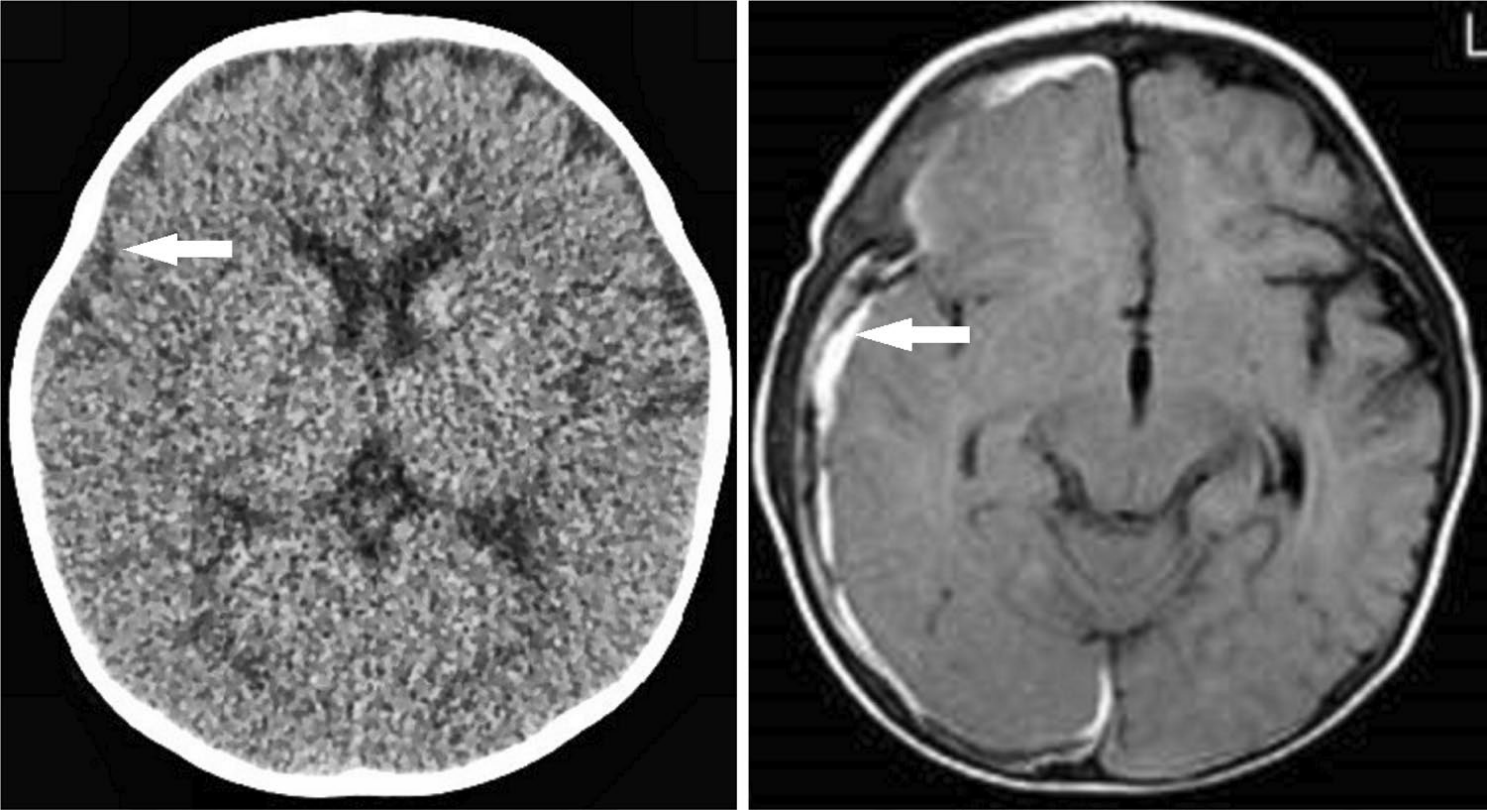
Case 4, male aged 6 months. Left: CT on arrival demonstrating thin, low-density SDH on the right side (arrow). Note that the density of the SDH is higher than that of the cerebrospinal fluid in the lateral ventricles and subarachnoid spaces on the left side. Right: MRI (T1-weighted image) 11 days after symptom onset demonstrating thin, high-intensity SDH on the right cerebral convexity (arrow). No mass effect or parenchymal abnormality was observed on the other sequences (not shown)
